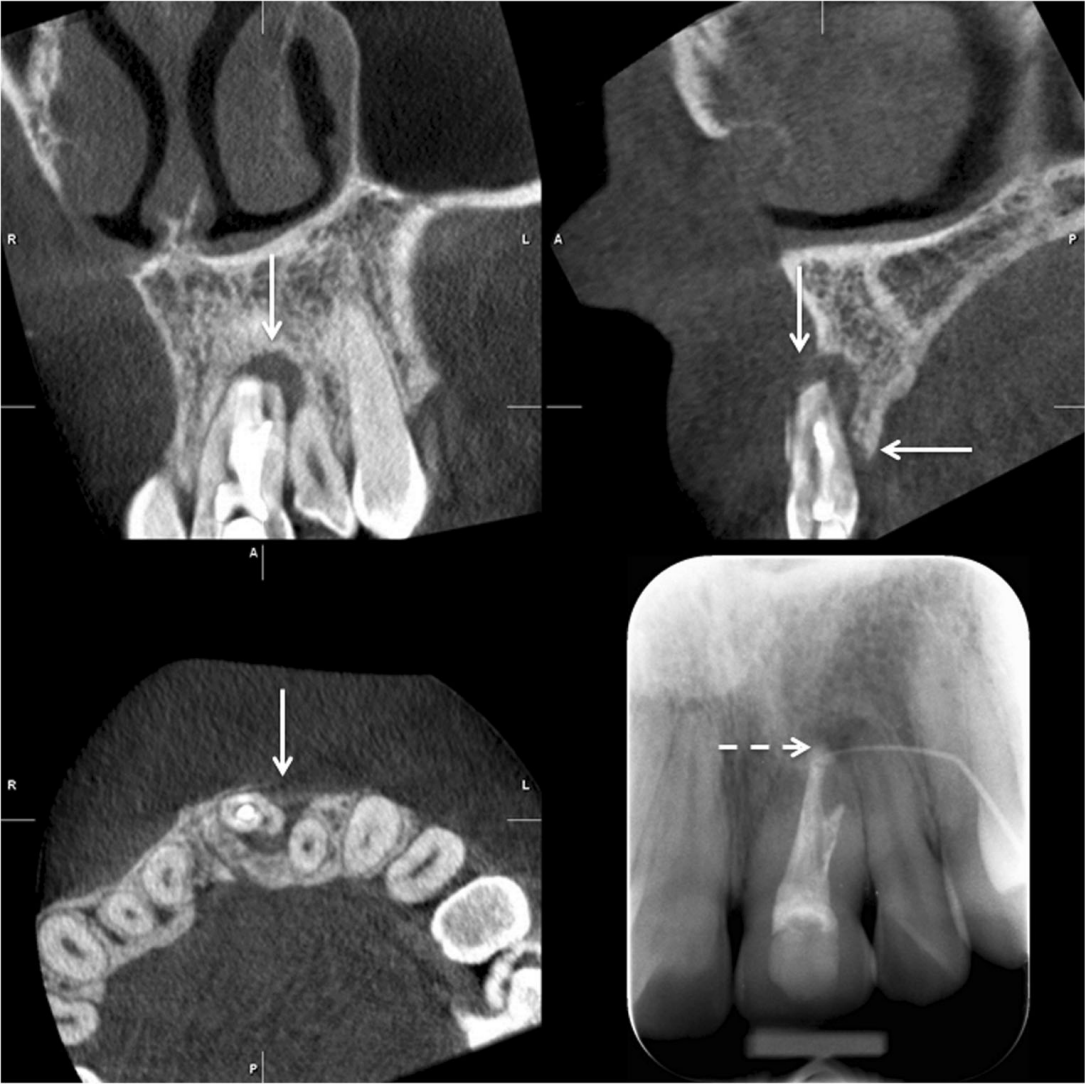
A deformed d. 21 was treated endodontically and resected several years ago. Clinically a fistula in the region d. 21 was found and fistulography with periapical radiography was done showing an apical periodontitis lesion (*lower right*). For the treatment planning a CBCT examination was done showing a large lesion indicating apical periodontitis with perforation of the labial cortex. Palatinally a vertical bone pocket is evident. Based on the CBCT findings extraction of the d. 21 was planned followed by implant treatment
